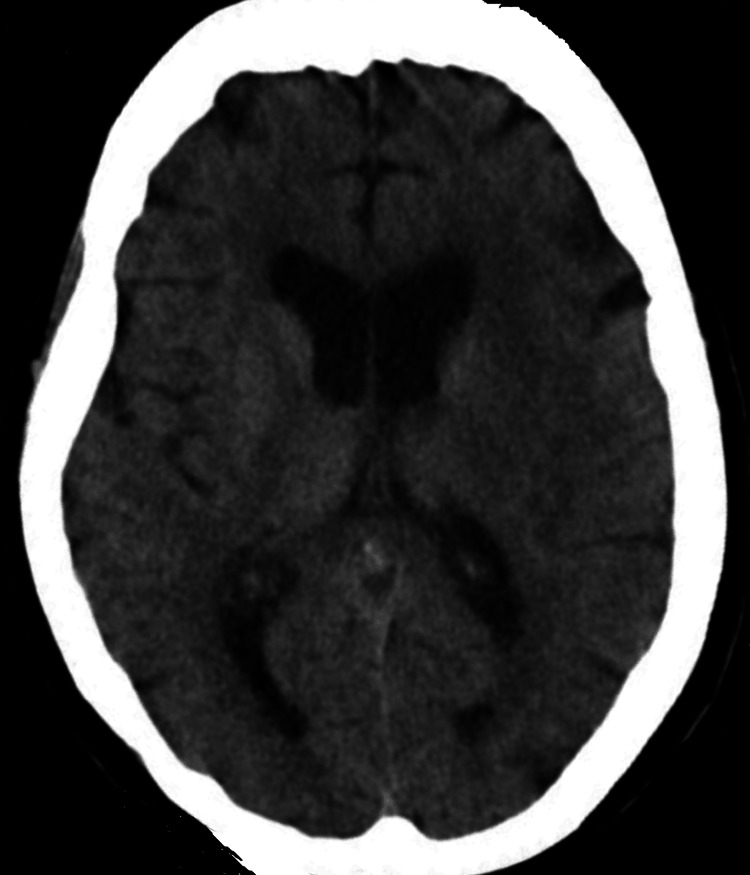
Repeat CT head showed no haemorrhage and no evidence of established infarct
